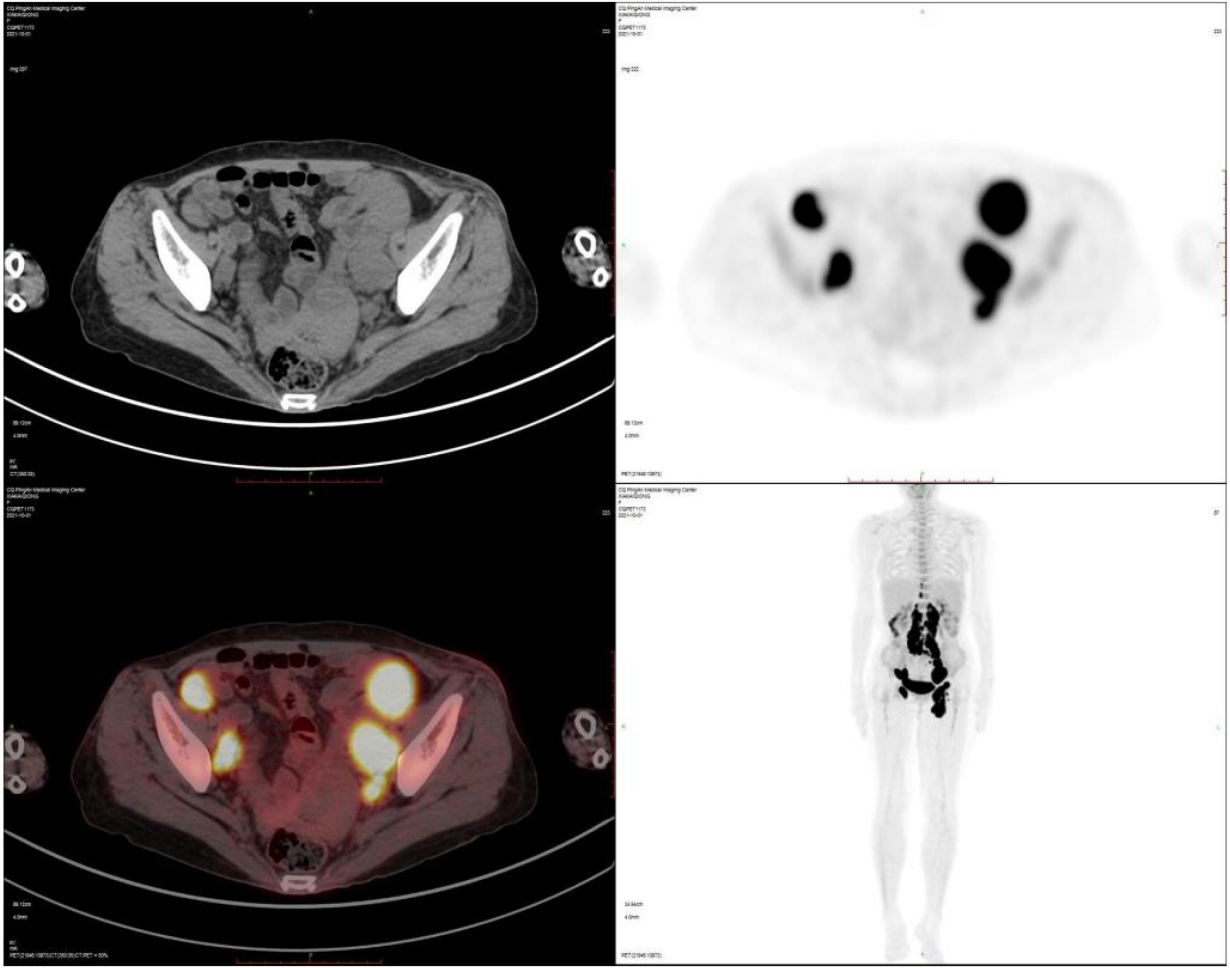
PET-CT before treatment: The scan reveals hypermetabolic lymph nodes (Deauville score 5) involving the bilateral inguinal, retrocrural, retroperitoneal, and pelvic regions.
